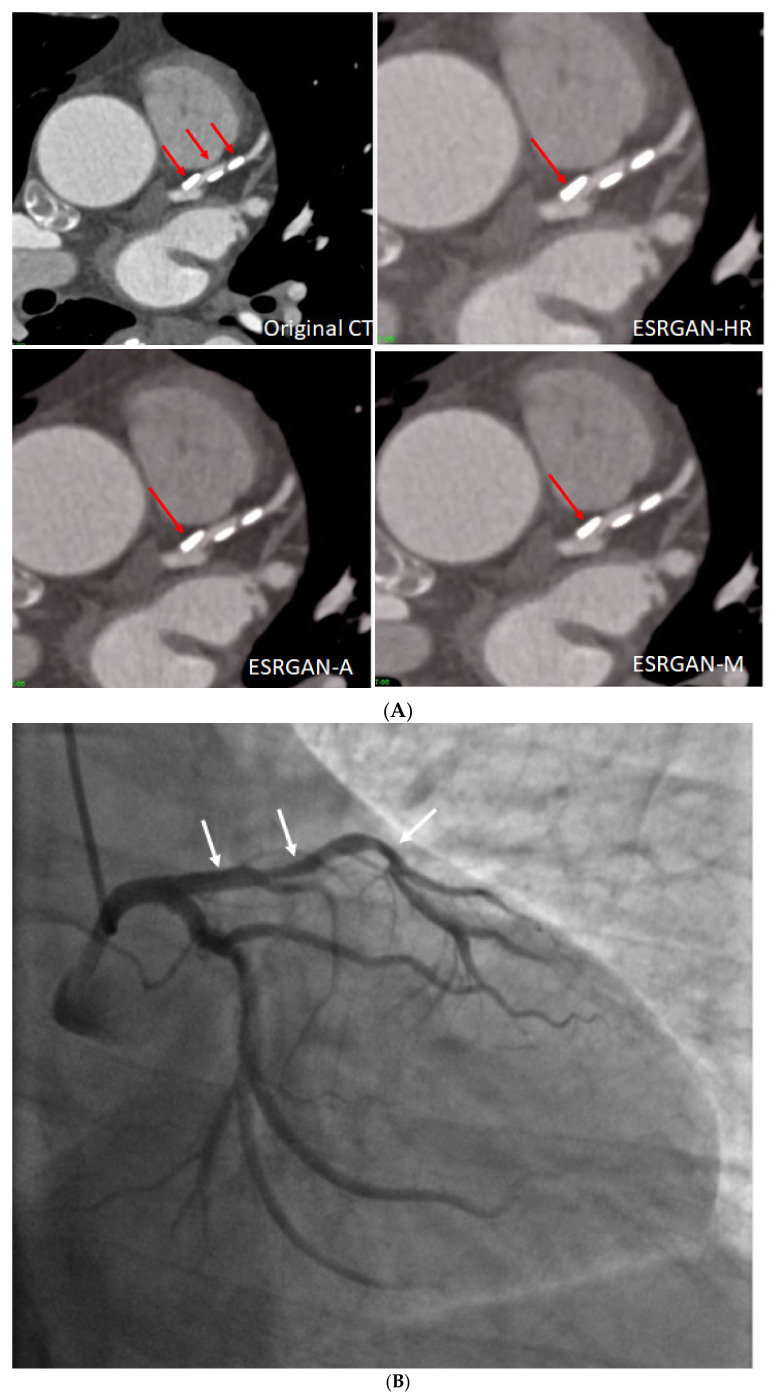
Multiple calcified plaques at the left anterior descending (LAD) artery in a 69-year-old man with coronary artery disease. (A): Comparison of the enhanced super-resolution generative adversarial network (ESRGAN)-processed images with the original coronary computed tomography angiography (CT) image in the assessment of coronary lumen stenosis caused by calcified plaques (arrows), with obvious improvement of lumen assessment, in particular, the ESRGAN-Median (ESRGAN-M) image in the assessment of the first plaque (long arrows). The measured mean stenosis was 63%, 44% and 86% at original CT, 58%, 39% and 83%, 56%, 37% and 83%, and 48%, 36% and 80% corresponding to ESRGAN-High Resolution (ESRGAN-HR), ESRGAN-Average (ESRGAN-A) and ESRGAN-Median (ESRGAN-M) images, respectively. (B): Invasive coronary angiography confirmed no significant stenosis at the LAD with corresponding diameters being 23%, 37% and 28% (arrows), respectively. ESRGAN-M images reduced the false positive rate by 33% when compared to the original CT and other two processed datasets.
